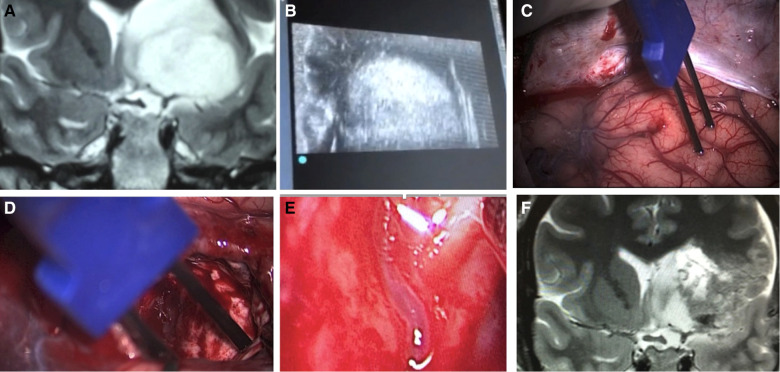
A case of insular glioma treated with LSTa as a resection limit. A - coronal T2-weighted MRI showing left-sided insular glioma with extension over the OC-IR line, suggesting a high probability of LSTa tumoral encasement; B - intraoperative echography as a tool to delineate the subcortical tumoral location; C - cortical brain mapping; D - subcortical brain mapping; E - intraoperative view of the LSTa within the tumor; F - postoperative MRI showing residual tumor beyond the OC-IR line, as it was the medial resection limit.
